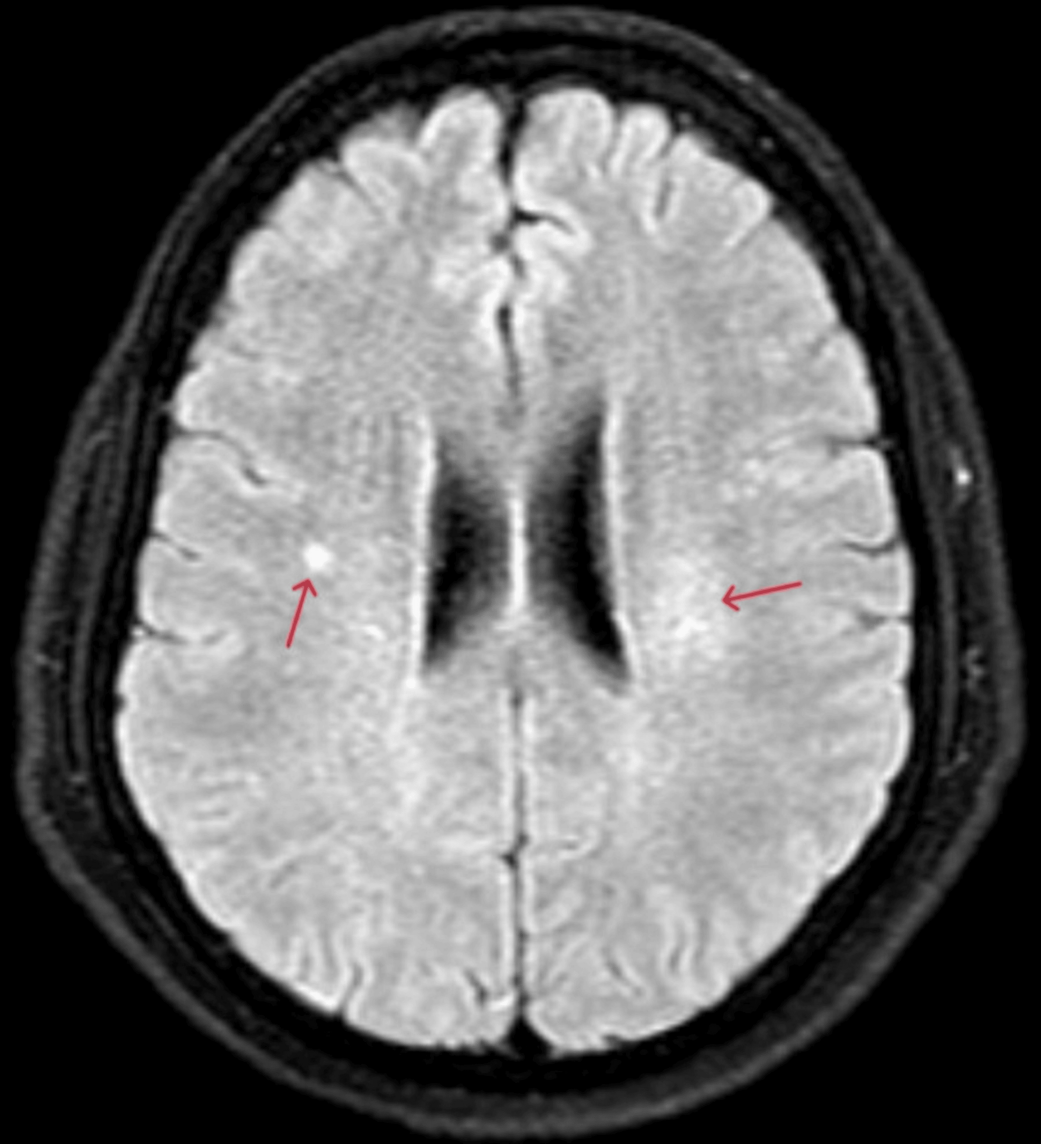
Axial FLAIR MRI of the brain showing the lesions. Small nodular hyperintensity on the right side and a diffuse hyperintense lesion on the left side (arrows). FLAIR: fluid-attenuated inversion recovery
